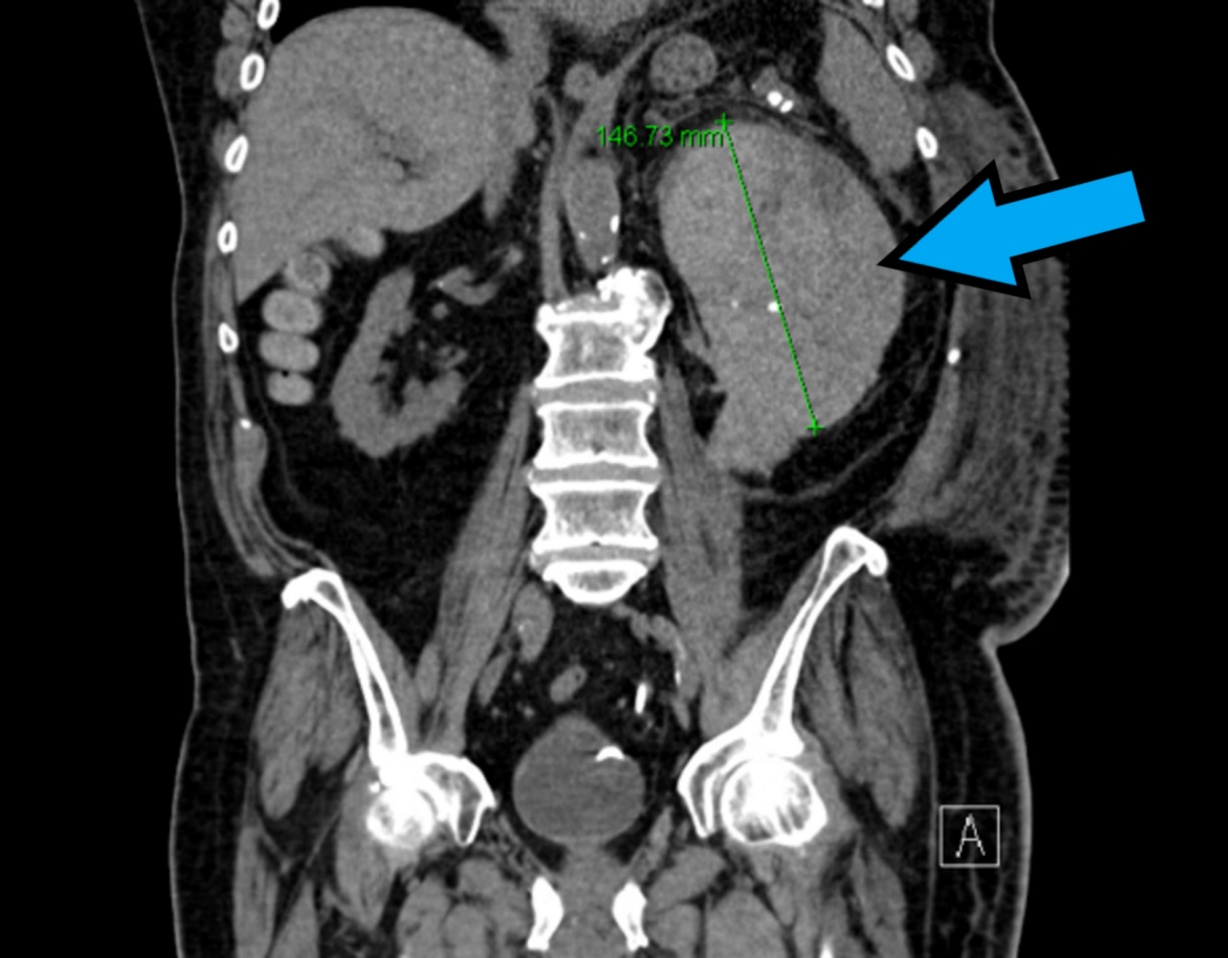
Computed tomography (CT) abdomen/pelvis showing left kidney hematoma (blue arrow)
